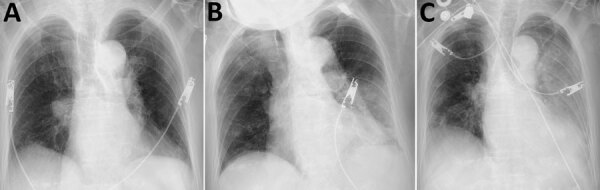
Chest radiographs (anteroposterior views) of hospitalized patient with coronavirus disease and fatal superimposed hypervirulent *Klebsiella pneumoniae* K2 sequence type 86 infection, Japan, 2020. A) Hospitalization day 1 (admission), showing no ground glass opacity and consolidation. B) Hospitalization day 7, showing asymmetric infiltrates with pleural effusion, mainly in left lung. C) Hospitalization day 8, showing infiltrate spread to right lower lung and worsened pleural effusion in left lung.
